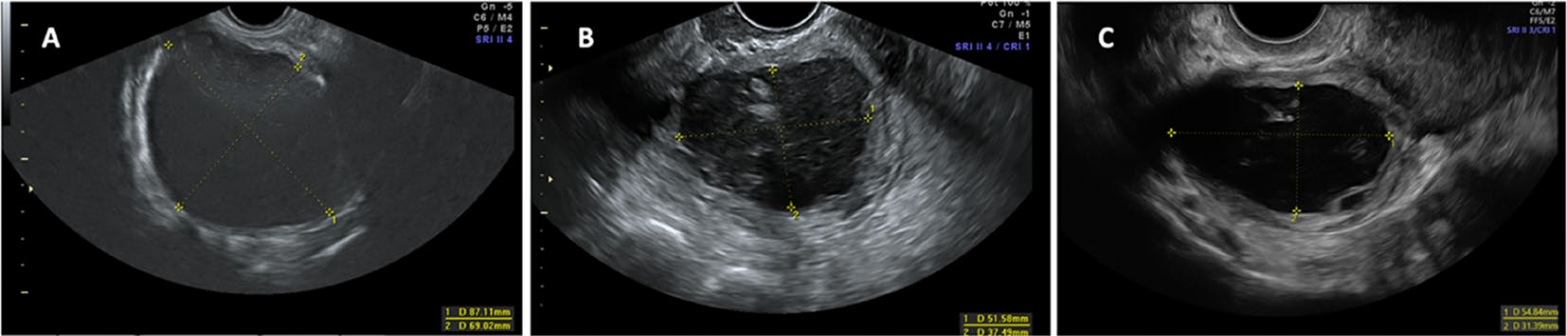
Sonographic evolution of ovarian endometrioma at baseline and at 12 and 24 months of follow-up. (A) Large endometrioma with a mean size of 78.0 mm which decreased to 44.5 mm at the 12-month follow-up in image (B) and to 43.1 mm at the 24-month follow-up in image (C).
